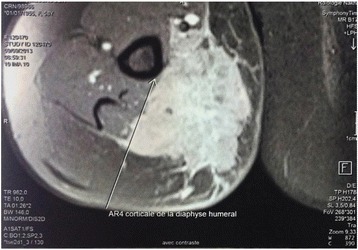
Contrast-enhanced, T1-weighted axial magnetic resonance imaging scan showing the process of the soft parts on the right arm
